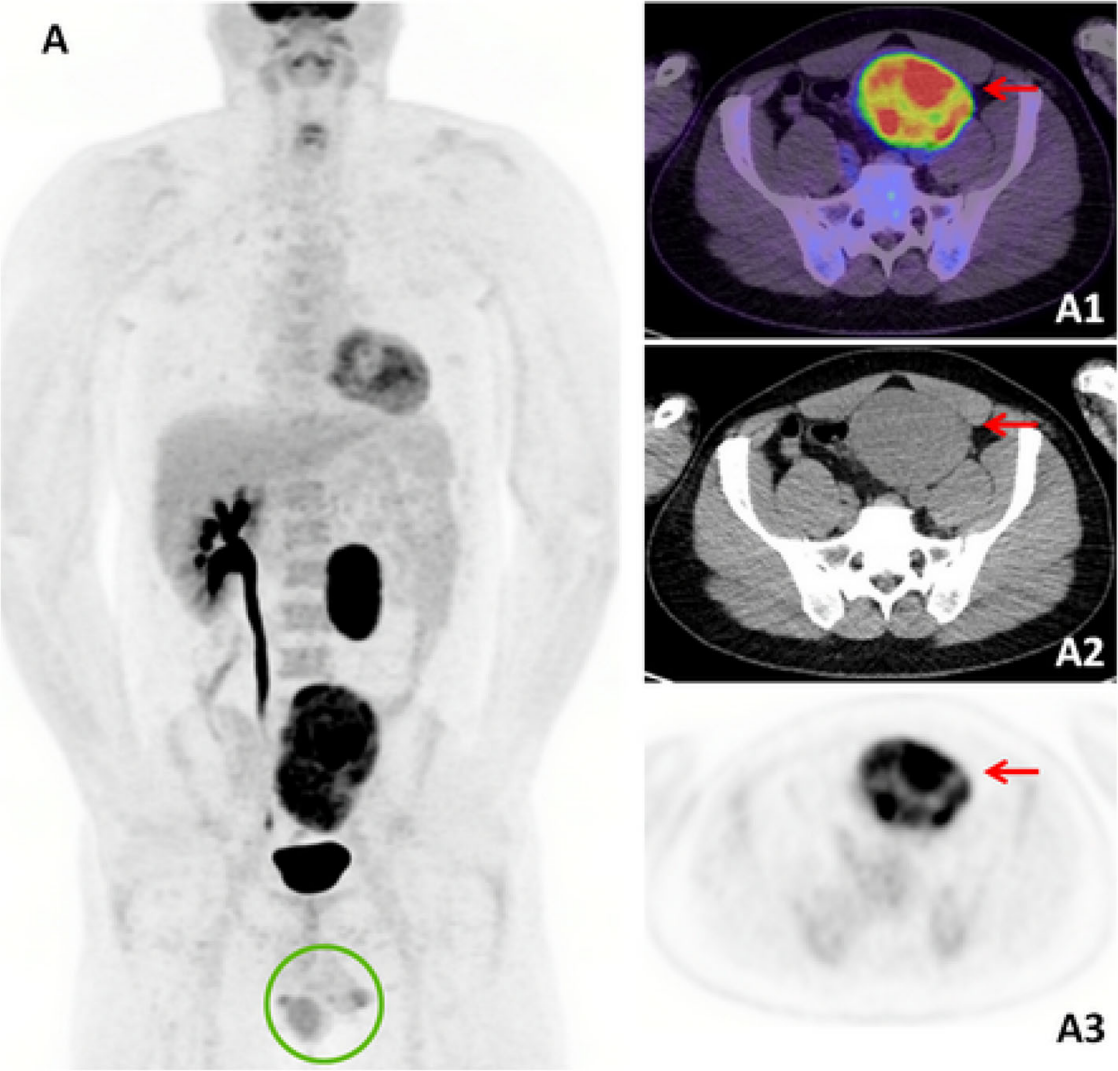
Case two - FDG-PET/CT maximum intensity projection (MIP) image demonstrates two large FDG-avid abdominopelvic masses, with absent left testicular scrotal activity [*circle*] (a). The dominant pelvic mass exhibits intense FDG-avidity (SUVmax 18.7) [*red arrow*]
